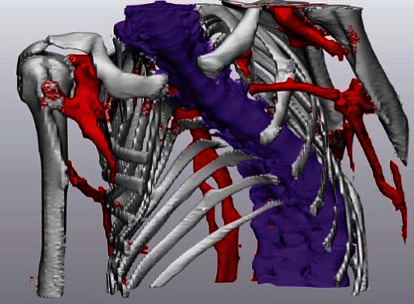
This image is a 3D model of patient 3's computed tomographic chest scan performed at the time of her initial presentation to our care. The sternum is absent to allow for visualization of the path of the spine, which is depicted in purple. Heterotopic bone formation is portrayed in red.
